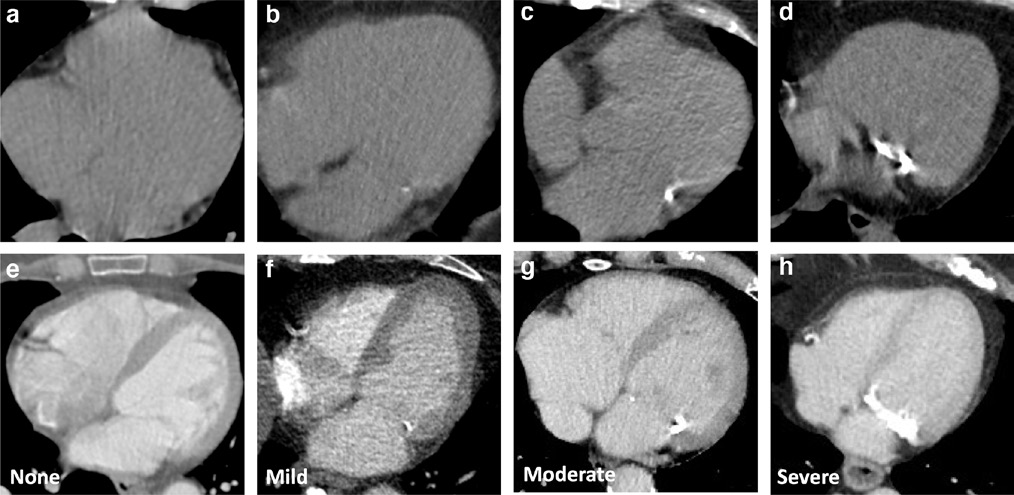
Mitral calcification severity. Images show different severity of mitral calcification from different patients on non-contrast (A–D) and contrast CT (E–H). Images show no calcification (A, E) and mild (B, F), moderate (C, G) and severe (D, H) calcification.
